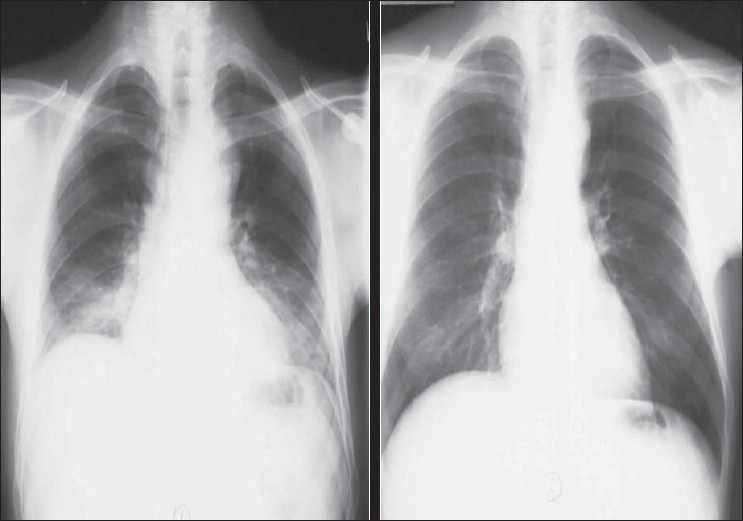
Chest radiographs on the same patient a few minutes apart showing the effect of technique; the left image shows mediastinal widening and basal clouding due to a poor inspiratory effort; the right image has been taken in good inspiration and looks entirely normal
